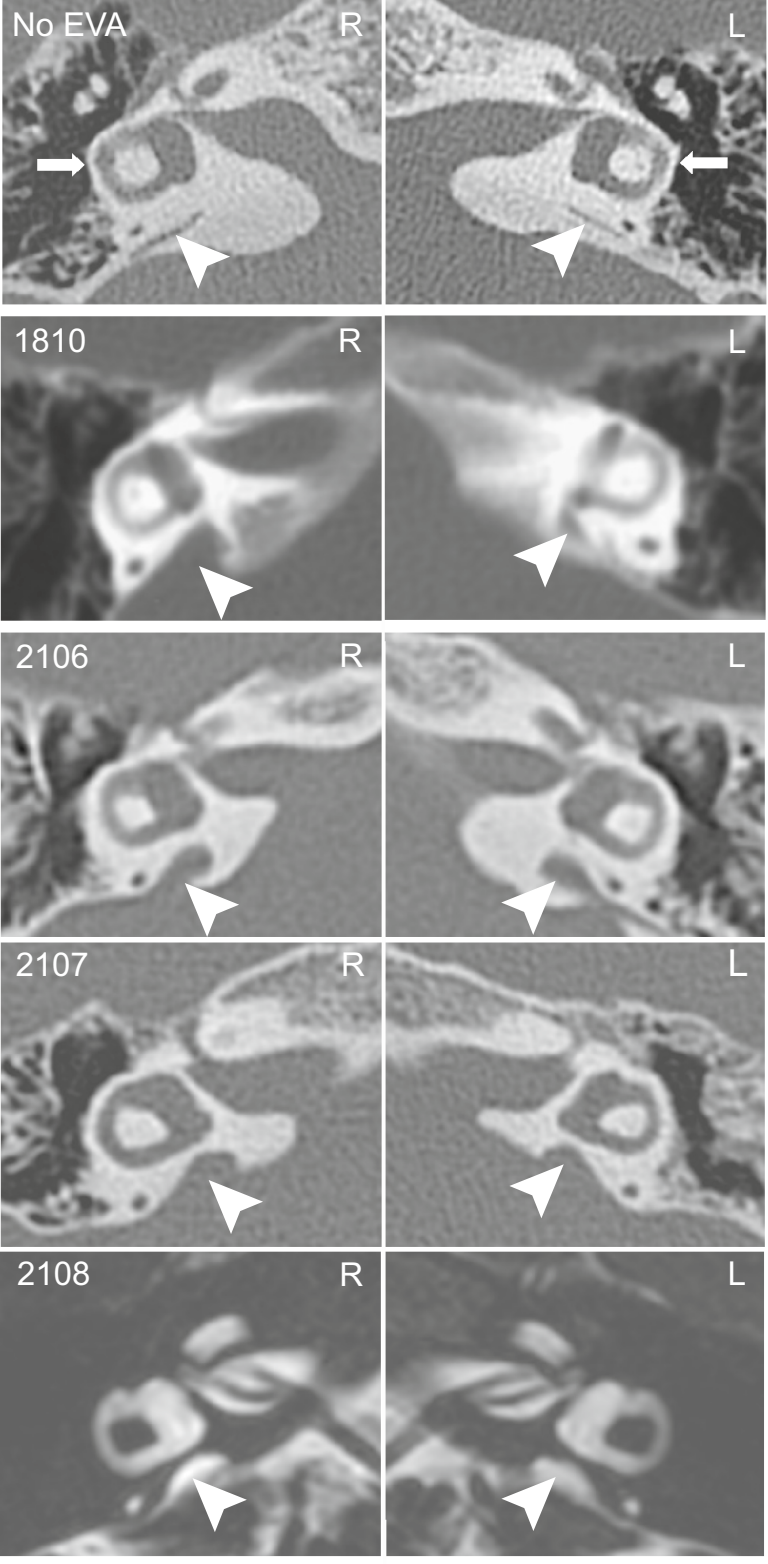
Radiological images of temporal bones of affected individuals with p.(Met1185Val) and p.(Gly1797Val) variants of *CHD7*. Montage of axial computed tomography (CT) (rows 1–4) and magnetic resonance (MR) (row 5) temporal bone imaging in a cohort subject with normal labyrinths and vestibular aqueducts (row 1), and from subjects with *CHD7* variant p.(Met1185Val) (row 2) and *CHD7* variant p.(Gly1797Ala) (rows 3–5). In row 1, normal anatomy is shown for reference, including the lateral semicircular canals (white arrows), which are often hypoplastic or absent in patients with CHARGE syndrome, and the normal vestibular aqueducts (white arrowheads) with a midpoint diameter less than 1 mm. For each patient (1810, 2106–2108), the lateral semicircular canals are well-formed, indistinguishable from normal (row 1, white arrow) and the vestibular aqueducts are enlarged (rows 2–5, white arrowheads) measuring 3 to 6 mm at the midpoint in each case. *R* right ear, *L* left ear
